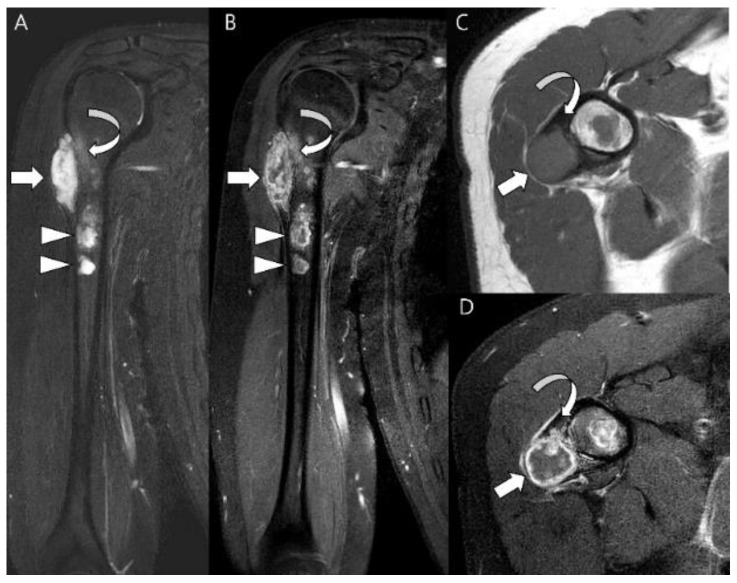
Secondary central chondrosarcoma of the humerus in a 19-year-old man. (A) Coronal T2-weighted image and (B) coronal T1-weighted enhanced images with fat suppression show multiple intramedullary chondroid tumors (arrowheads) with peripheral and septal enhancement in the humerus, suggesting enchondromatosis. The major lesion shows bone expansion at the metaphysis (arrow) with peritumoral edema and enhancement (curved arrow). (C) Axial T1-weighted and (D) enhanced images show a peripherally enhancing major lesion (arrow) with cortical remodeling (curved arrow).
